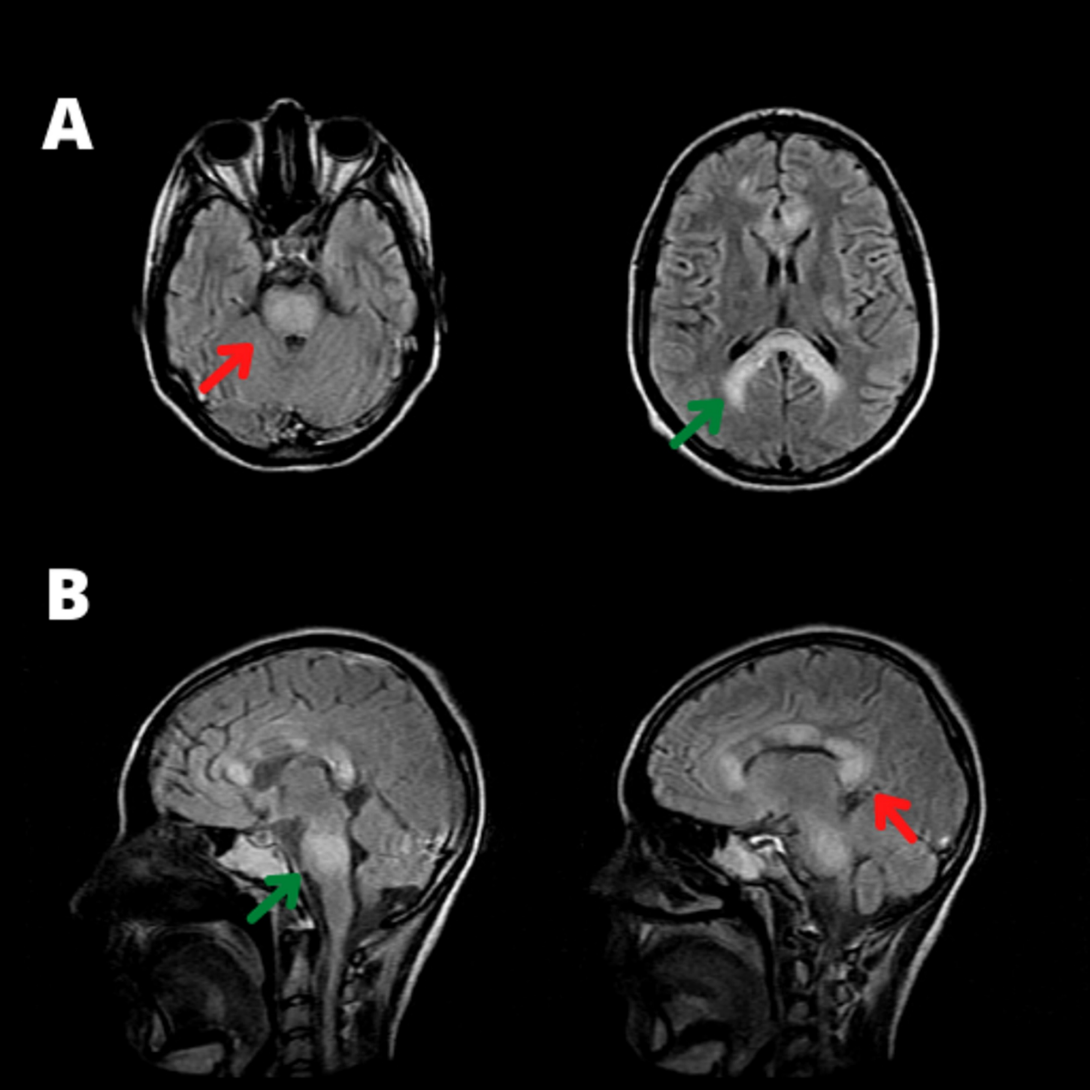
FLAIR sequences of axial (A) and sagittal (B) planes demonstrate the usual pattern of demyelinating lesions of the white matter with a predominance of lesions in the brainstem (green arrows), as well as in the posterior aspect of the corpus callosum and internal capsule (red arrows). FLAIR: fluid attenuation inversion recovery
